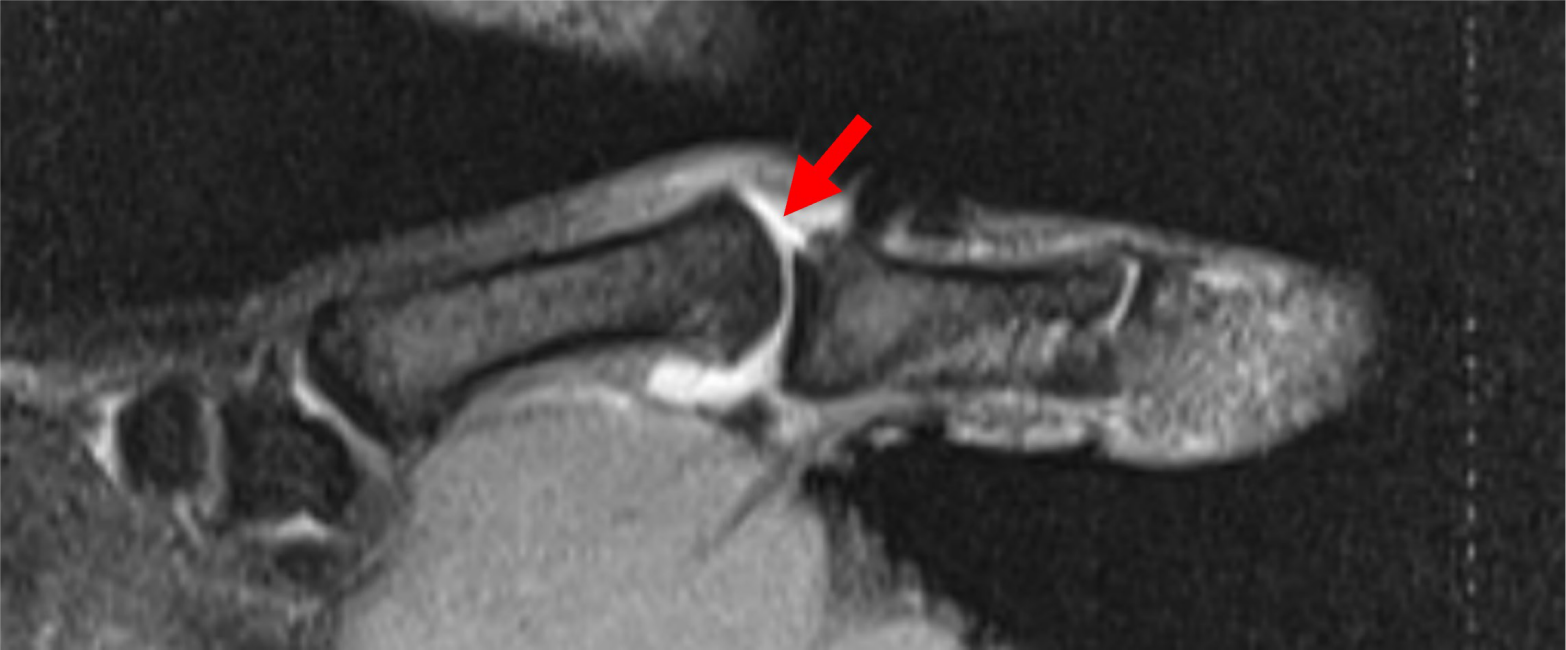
The sagittal magnetic resonance imaging scan shows chronic ulnar collateral ligament tear of the first metacarpophalangeal joint. Note that volar subluxation of the joint secondary to tear of the dorsal joint capsule (red arrow).
